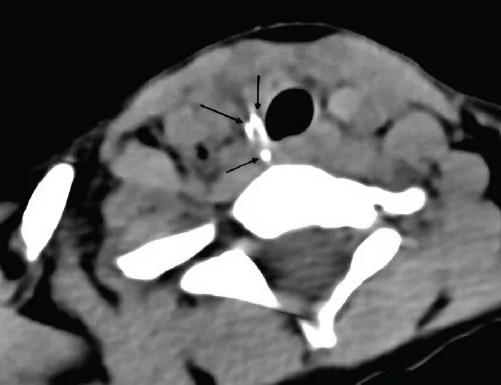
Axial view of a noncontrast computed tomography depicting glass foreign bodies in the neck of a 3-year-old male (arrows).
